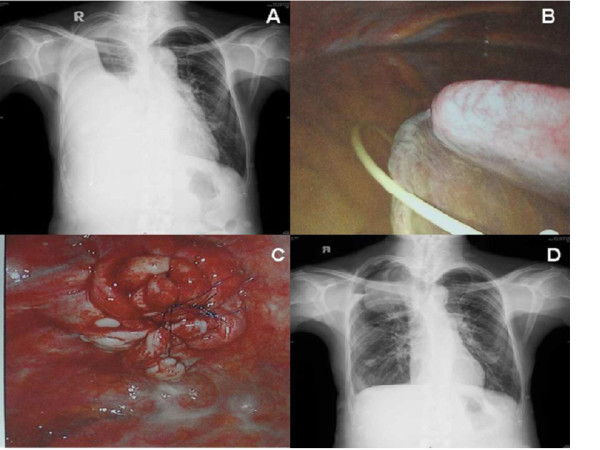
Chest plain radiograph after thoracoscopic plication. (A) Right massive and loculated pleural effusions were seen. (B) Massive pleural effusion and dislocation of cystoperitoneal shunt were found under thoracoscope examination. (C)The meningocele was reduced to a lump of soft tissue at the root with multiple plication. (D) Postoperatively chest radiograph at 2 months showed a marked improvement in lung volume.
